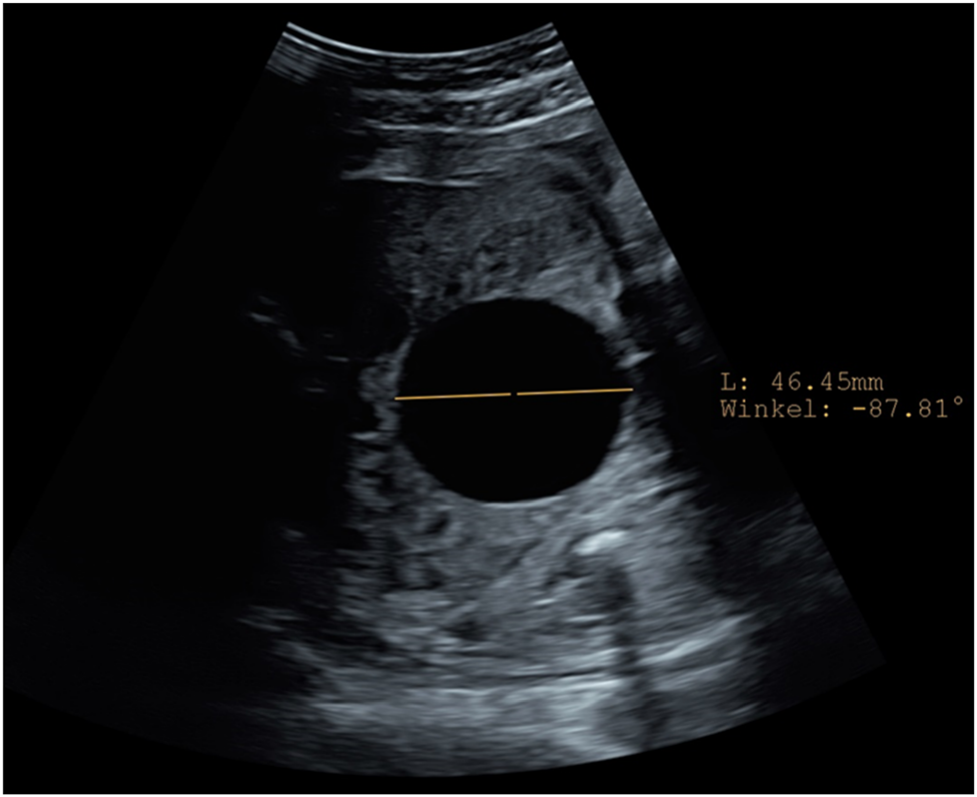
Two days after introduction of opioid treatment ultrasound showing an isolated enlarged fetal bladder (diameter of 47 × 40 mm) with thin walls.
